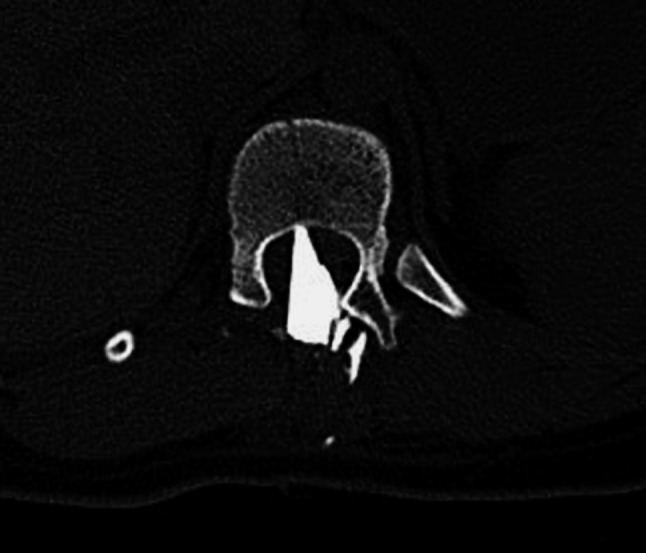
CT scan Th11–Th12. A piece of glass window penetrating to the spinal canal
